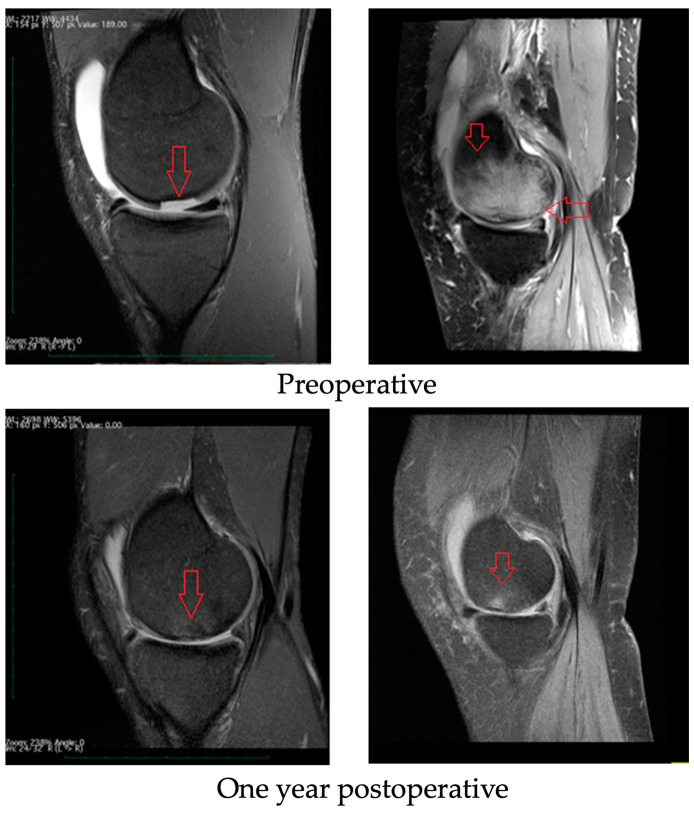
MRI findings: preoperative and one year postoperative. Red arrows for preoperative images show cartilage damage with ICRS grade IV loss (image on the left) and marrow oedema as a result of cartilage damage and lack of treatment (image on the right) and for one year postoperative images red arrows show evolution of cartilage healing (image on the left) and withdrawal of oedema from bone (image on the right).
